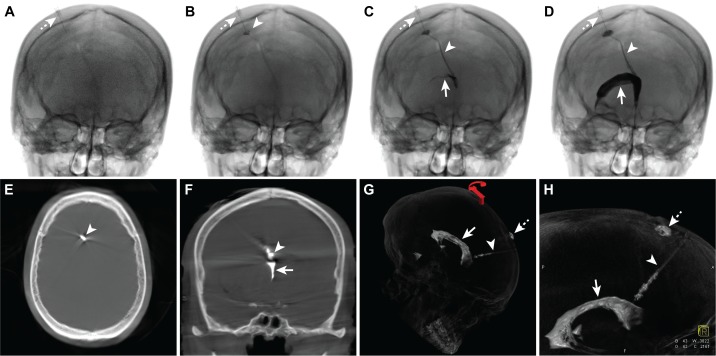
Fluoroscopic images during contrast injection of the Ommaya reservoir and flat panel computed tomography images after contrast injection. Serial fluoroscopic images (A-D) obtained during iodinated contrast injection of the Ommaya reservoir demonstrate the needle in position within the reservoir (A-D, dashed arrow) prior to injection (A) and during injection (B-D). There is progressive contrast opacification of the Ommaya reservoir (B, arrowhead), tubing (B, C, arrowhead), and right lateral ventricle (D, arrow), but no contrast extends into the subdural space. (E-H) Three-dimensional images reformatted from a flat panel computed tomography study after Ommaya reservoir contrast injection. There is no contrast evidence within the right frontal subdural collection (E, F). The Ommaya tubing is well seen with contrast within it (E, F, arrowhead), and contrast is noted filing the third ventricle on the coronal image (F, arrow). Additional reformatted images (G, H) demonstrate contrast filling the Ommaya reservoir (G, H, dashed arrow), tubing (G, H, arrowhead), and right lateral ventricle (G, H, arrow). No contrast extends outside of the Ommaya reservoir or ventricle system into the subdural space.
